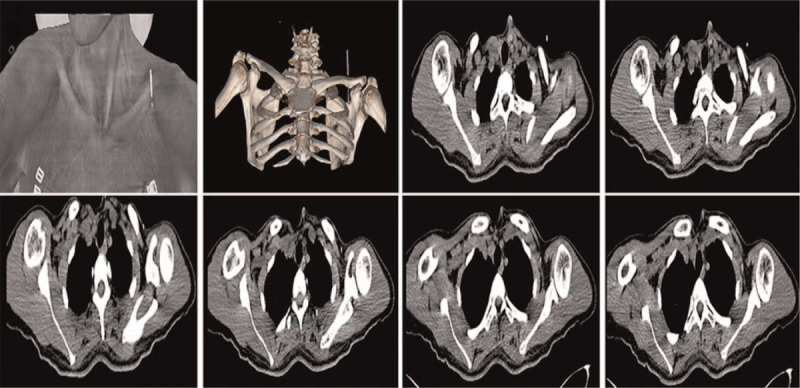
Position relationship between acupuncture points and pleural cavity on computed tomography.
